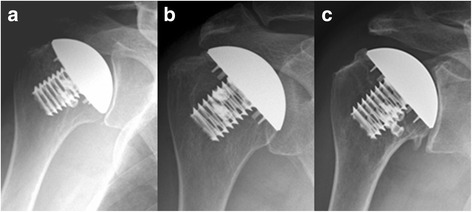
Anteroposterior radiographs of a seventy-two-year-old woman made immediately after hemi shoulder arthroplasty (a), at the short-term follow-up showing no radiolucency or osteolysis (b), and at the mid-term follow-up showing osteophyte formation superior around the humeral head implant and inferior (c)
